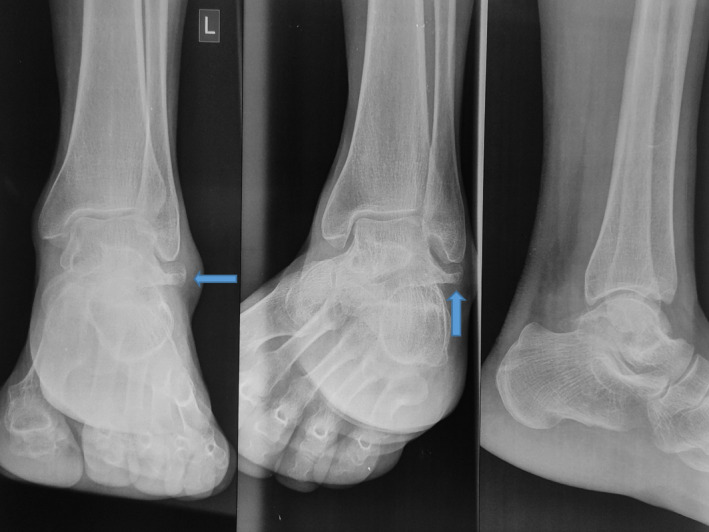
Radiographs of ankle showing bony outgrowth from lateral aspect of talus just below the lateral malleolus (arrow)
